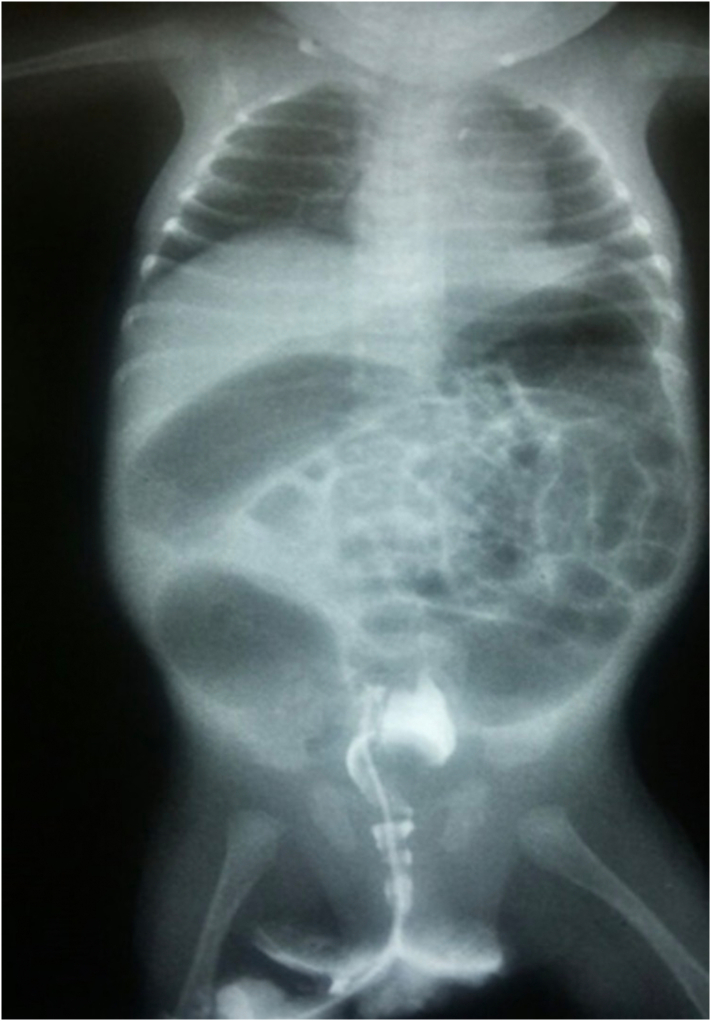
Contrast enema X-ray: A contrast enema showed resistance to the passage of the rectal tube and contrasted pooling in the rectosigmoid region (extravasation).
